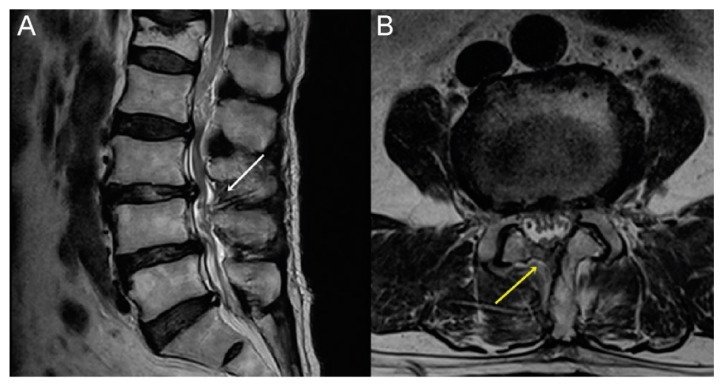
Radiological outcome after endoscopic suturing. (A) T2-weighted sagittal (white arrow: suture site) and (B) T2-weighted axial magnetic resonance images (yellow arrow suture site). There is no evidence of residual cerebrospinal fluid.
